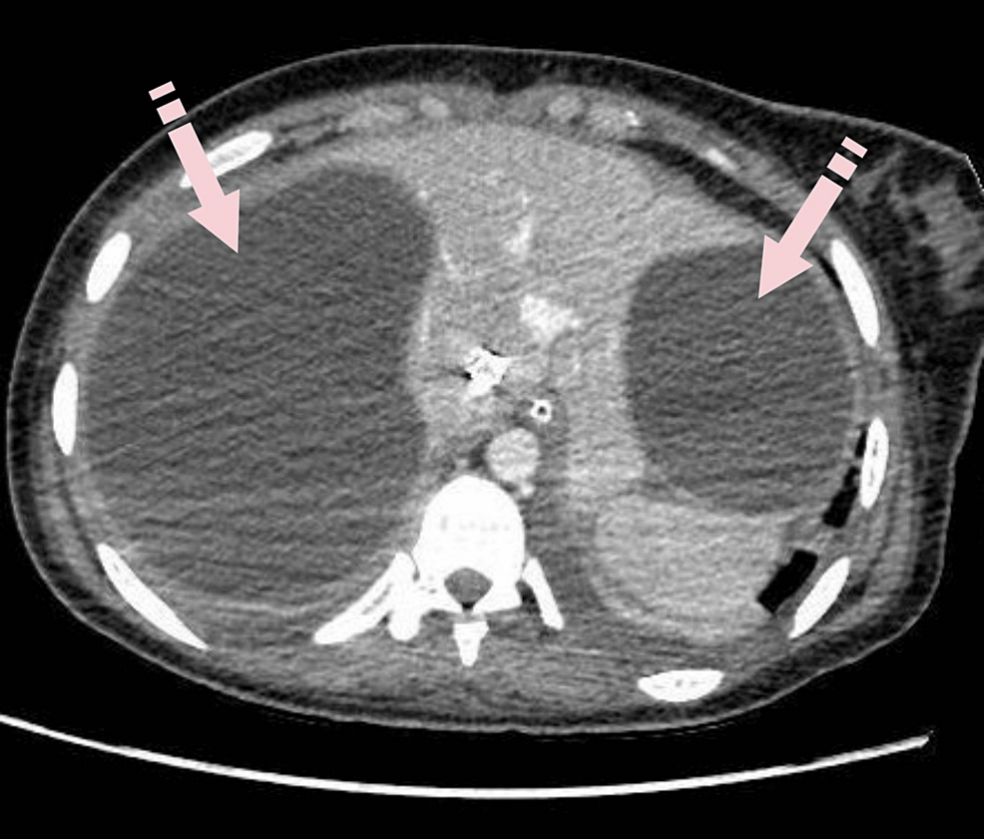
CT of the abdomen with contrast, axial plane: two subcapsular hepatic cysts compressing the right and the left hepatic lobes.
